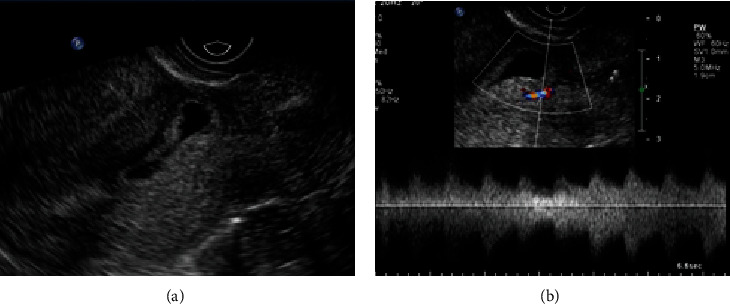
A 36-year-old female (gravida 4, para 1) presented with amenorrhea for 47 days. (a) The sagittal grayscale image showed that the sac was implanted in the lower endometrial cavity, protruding into the scar. (b) The color Doppler image showed the trophoblastic blood flow from the lower posterior uterus. Chorionic villi were not visible inside the scar with a laparoscopy.
